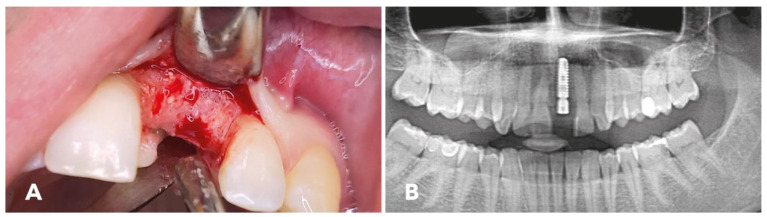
(A) Reconstructed alveolar ridge at re-entry 6 months after augmentation surgery; cerabone^®^ granules surrounded by bleeding bone are visible superficially. (B) Postoperative panoramic radiograph with inserted implant.
